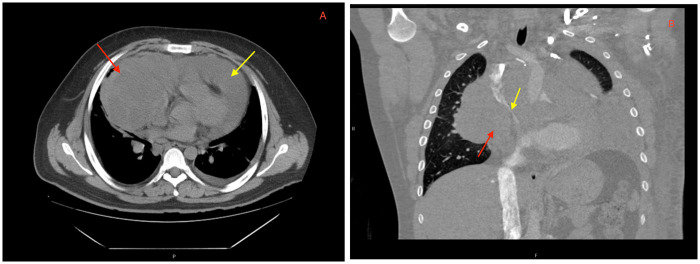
CT imaging showing a (A) large mediastinal mass (red arrow) and pericardial effusion (yellow arrow) on presentation. Repeat CT angiography post-pericardiocentesis (B) redemonstrating a mediastinal mass (red arrow) abutting the right atrium and ventricle with significant narrowing of the superior vena cava (yellow arrow).
